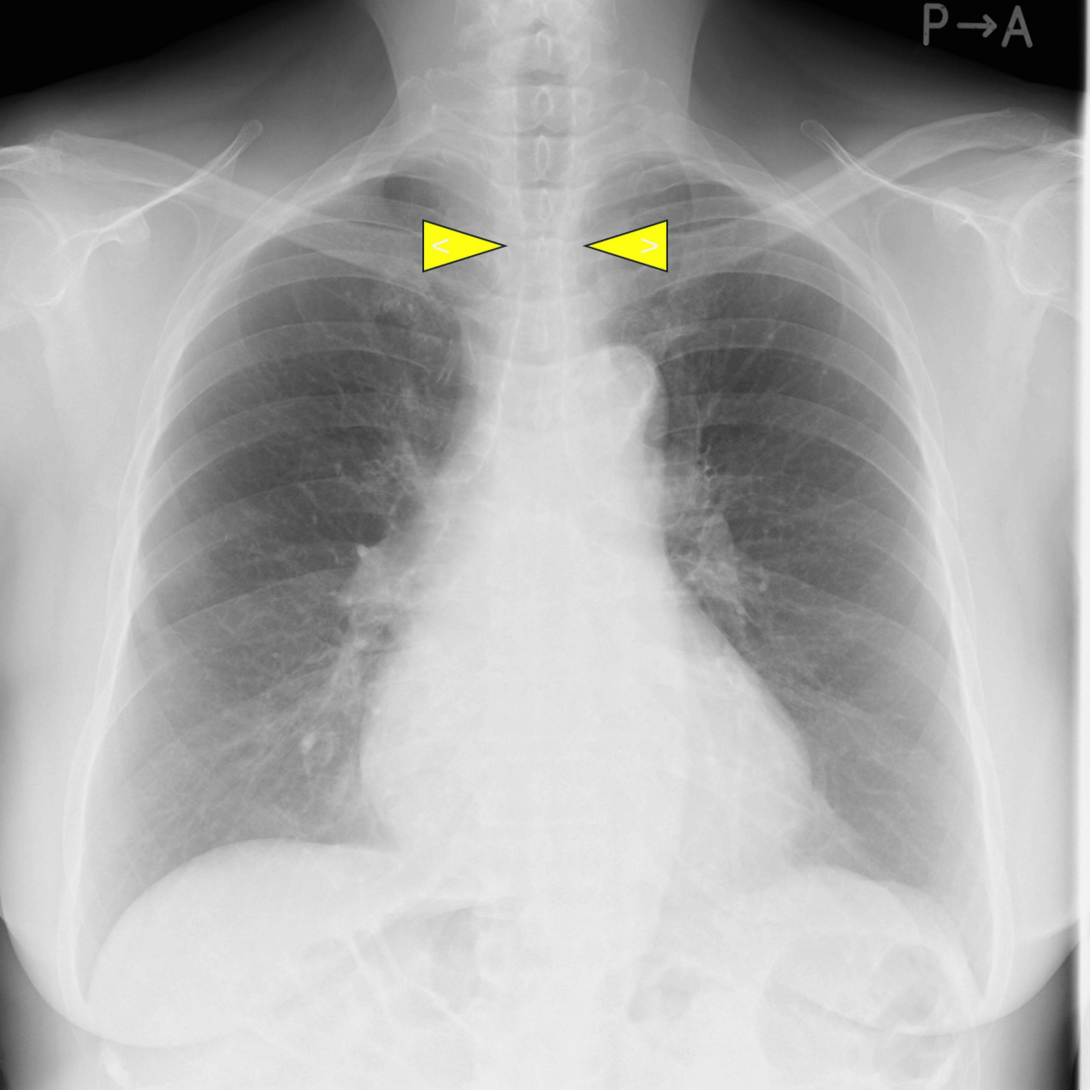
The preoperative chest X-ray image of the present case. A slight narrowing of a portion of the trachea (yellow arrows) is observed.
